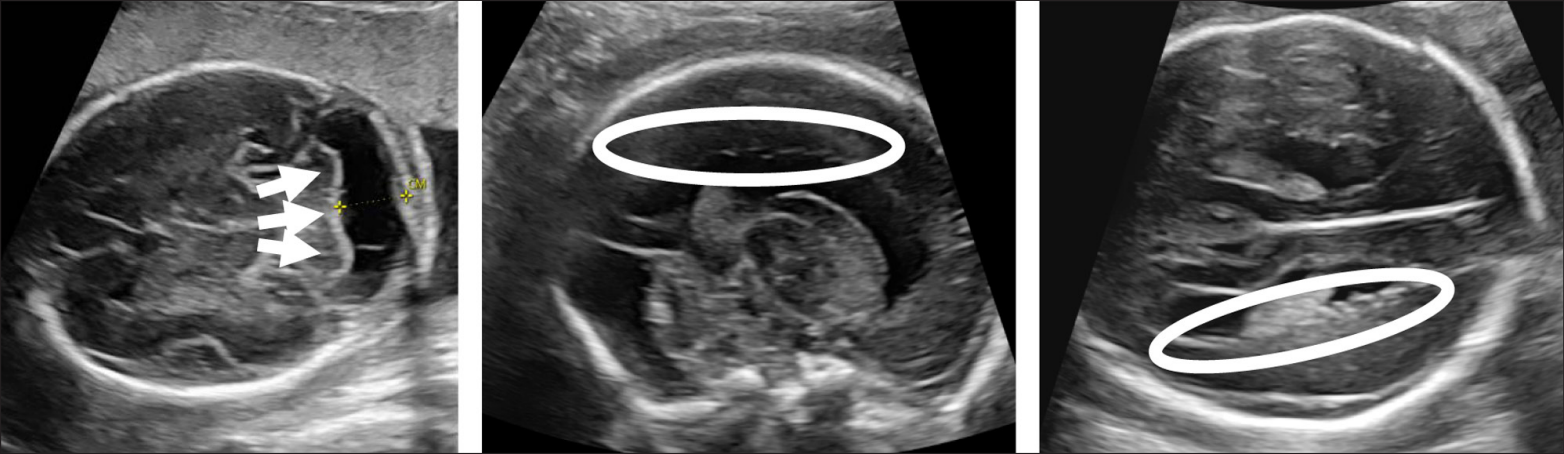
Prenatal ultrasound images at 25 weeks of gestation demonstrate a dilatation of the cisterna magna >10 mm (arrows) and nodular lining of walls of the lateral ventricles (oval).
